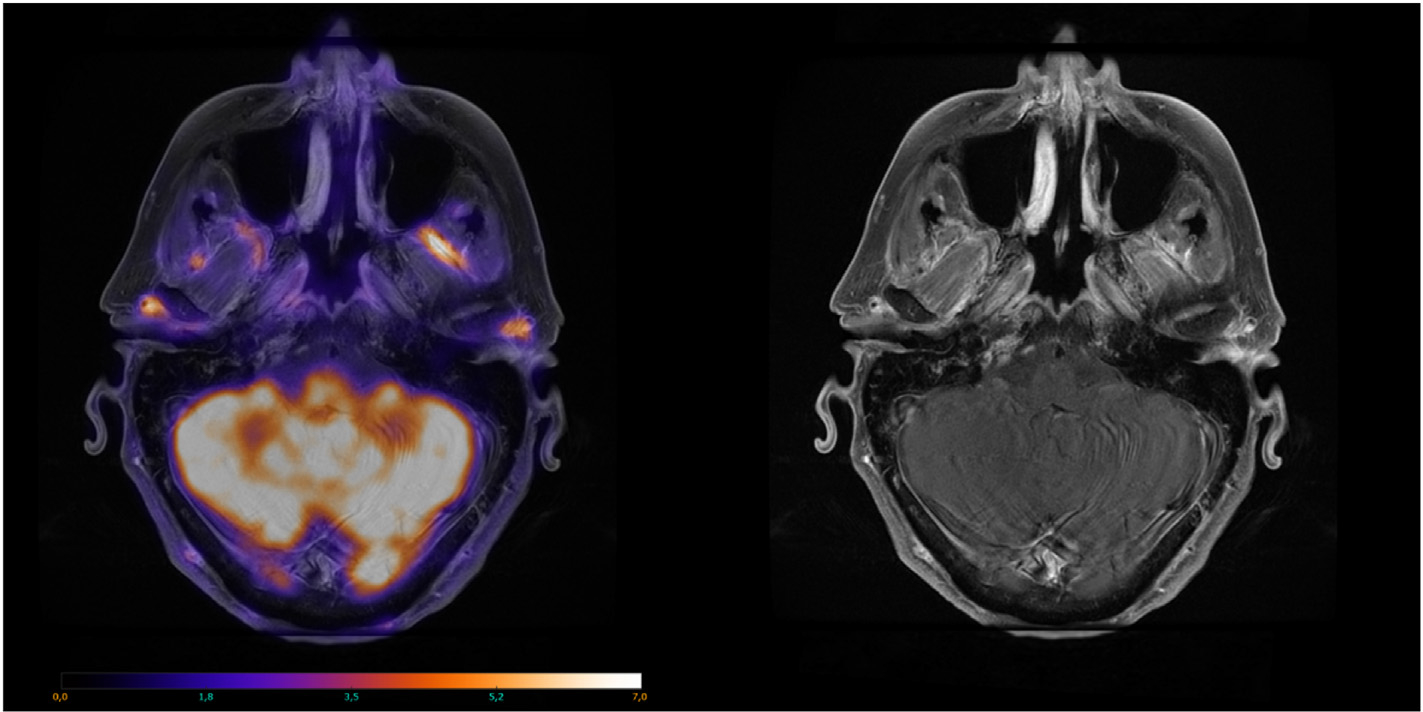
Left: Axial FDG-PET/MR image of a patient with cGCA. Increased uptake can be observed here in the common superficial temporal arteries, maxillary arteries, right posterior deep temporal artery, and right occipital artery. Right: Axial contrast-enhanced T1-weighted MR image in the same patient without FDG-PET overlay. Concentric vessel wall thickening and attenuation can be observed here in the right common superficial temporal artery and right occipital artery. Image courtesy of Lars Gormsen, MD, PhD, Aarhus University Hospital (Denmark). FDG-PET/MR, [18F]-fluoro-2-deoxy-d-glucose positron emission tomography/magnetic resonance; cGCA, cranial GCA.
